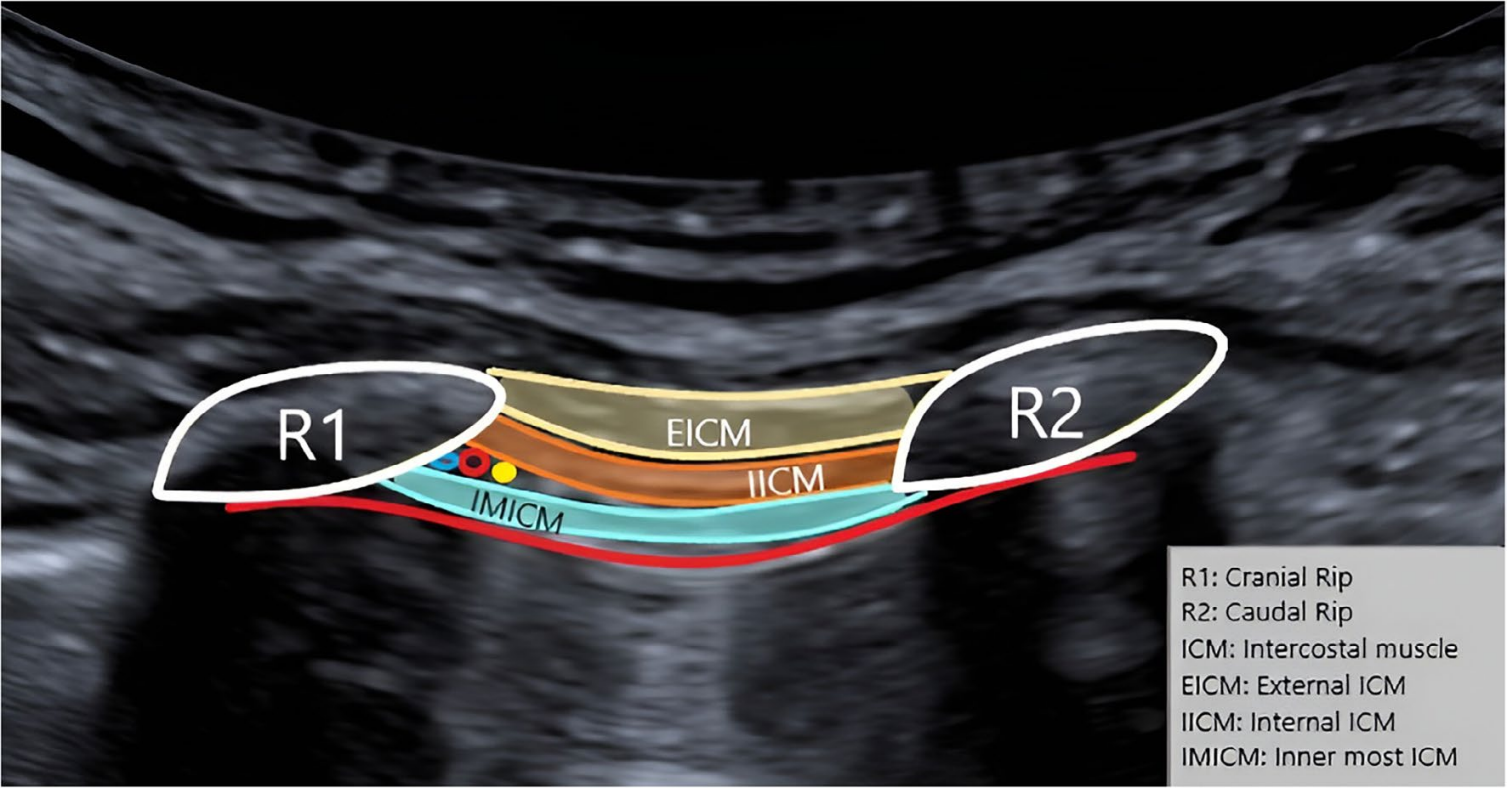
Identification of the different layers of the intercostal space on ultrasound.
